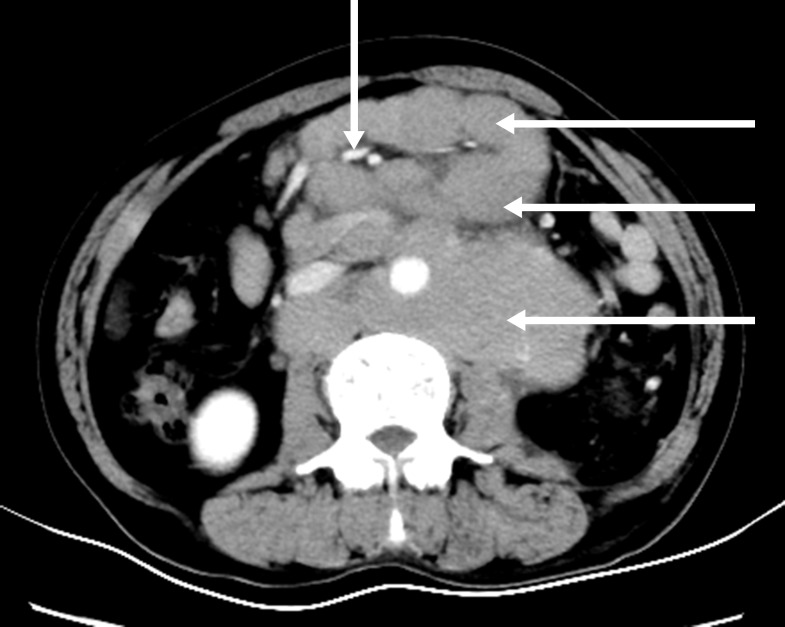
Contrast abdomen computed tomography shows multiple mesenteric and retroperitoneal lymph node enlargement and integration into masses with mild-middle enhancement, the mesenteric vessels embedded (sandwich sign)
